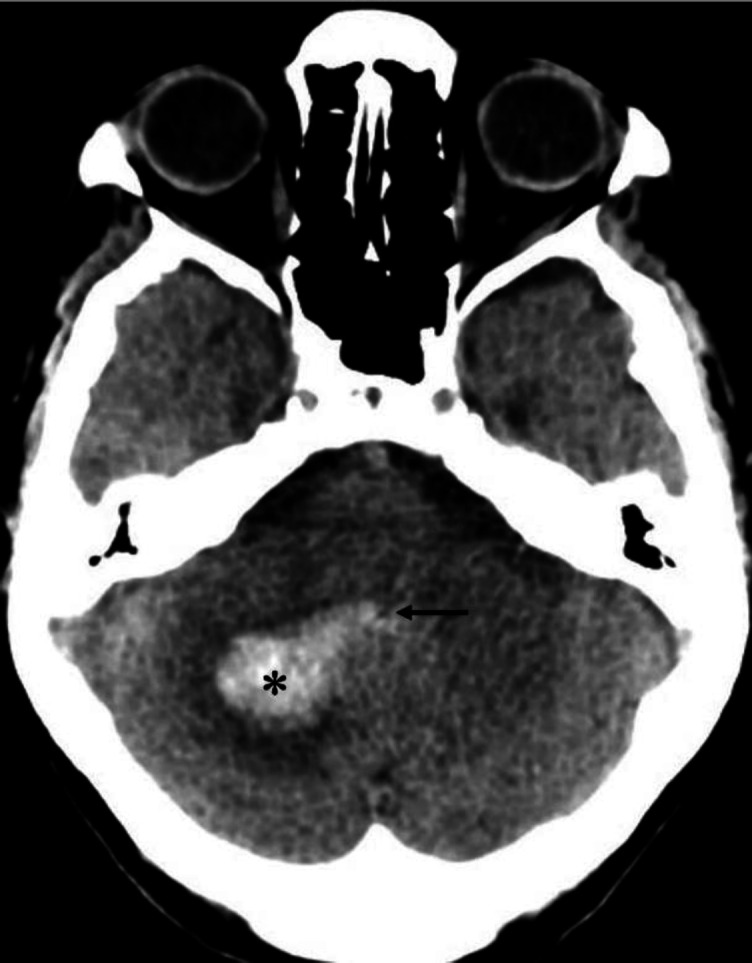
Non-contrast CT brain of a 91 year-old female with uncontrolled hypertension shows a hyperdense region in the right cerebellum (asterisk), corresponding to an acute haemorrhage. Note the intraventricular extension of the haemorrhage into the 4th ventricle (black arrow)
